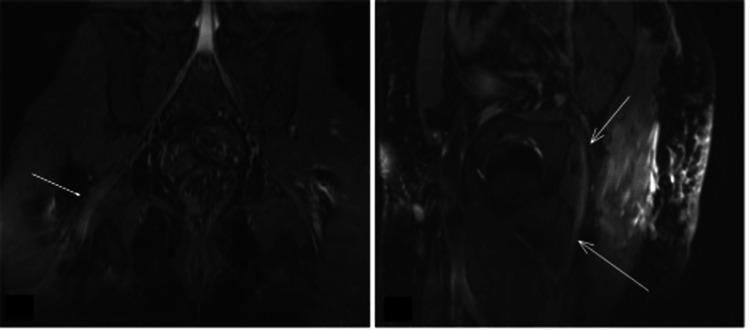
MRI sciatic neurography showing T2 hyperintensity in the right sciatic nerve
